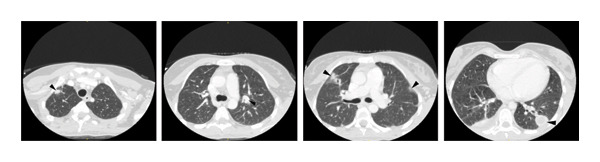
CT‐scan (axial view) in which downsizing of the lung abscesses is observed (black arrows).
